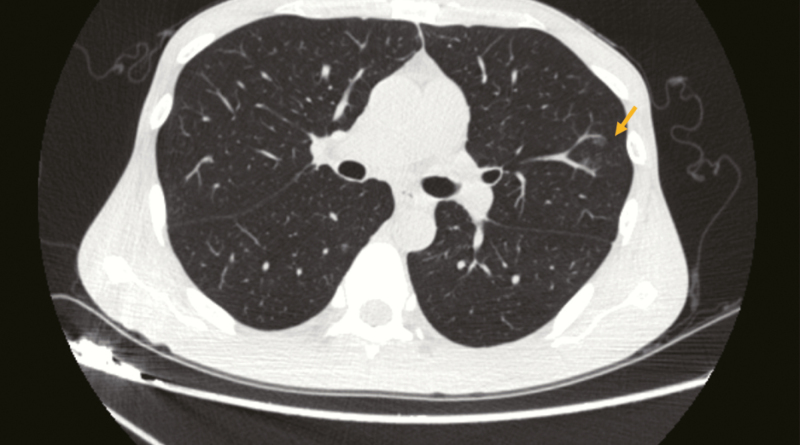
The chest CT manifestations of patient 1 showing plaques and exudation.
